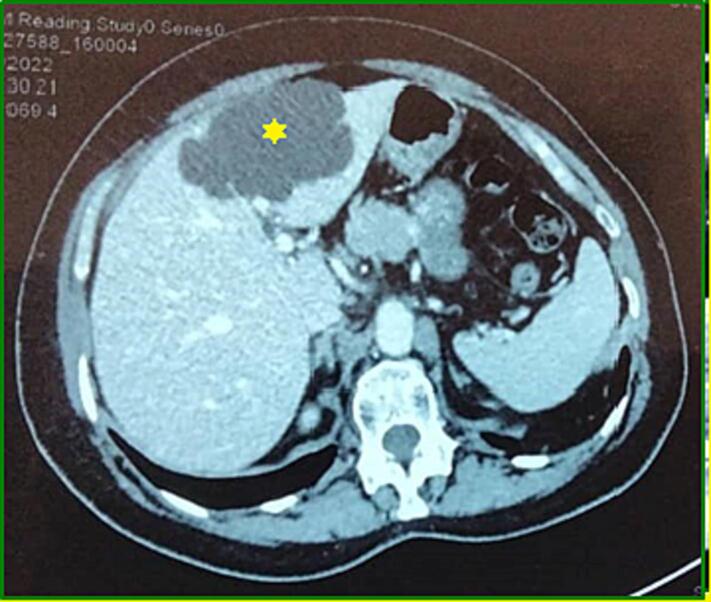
Axial section of an injected CT revealing a fluid-dense hepatic cystic lesion that does not enhance after contrast injection (*).
